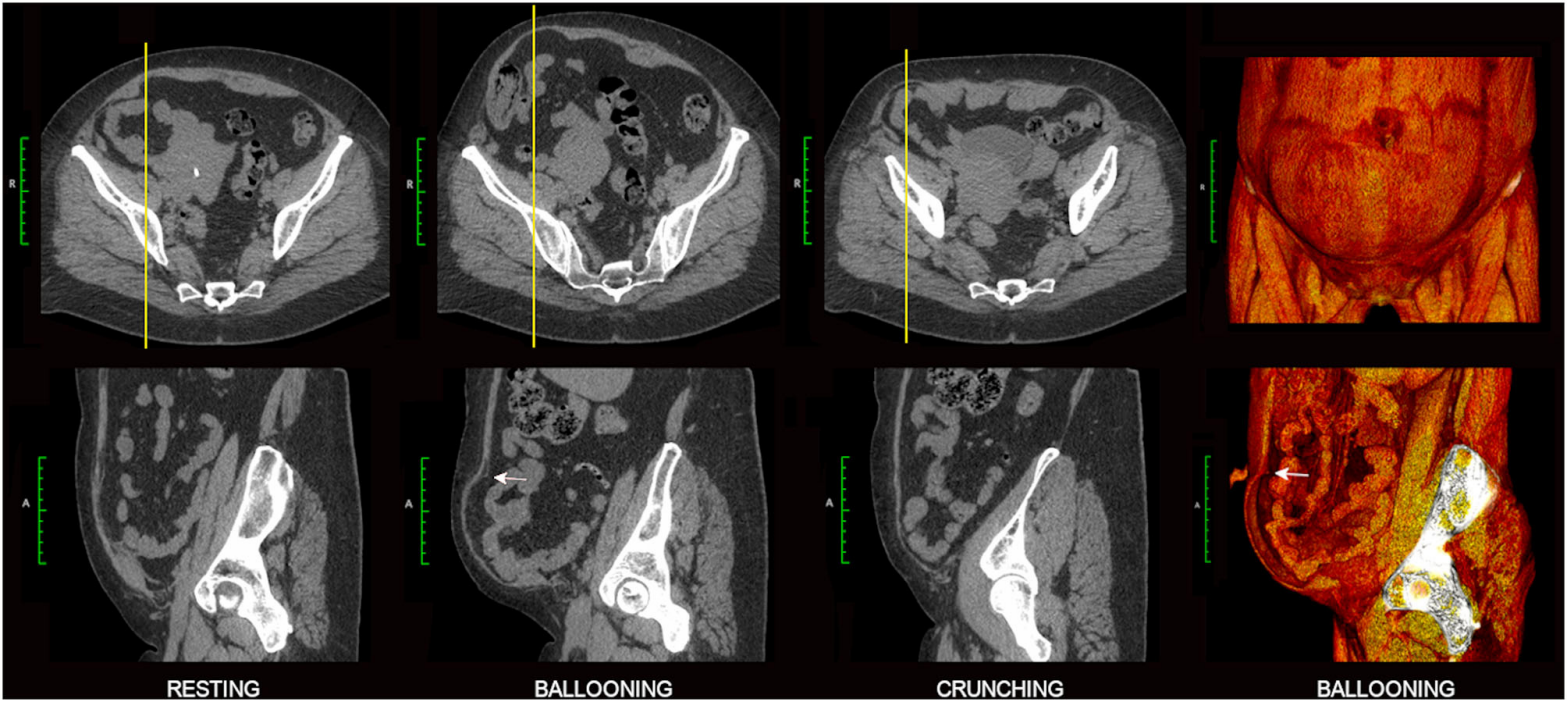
Value of ballooning CT acquisition. Axial and sagittal 2D images are shown from a 3-phase functional CT examination of a 50 year old female with broad-based right lower quadrant incisional hernia. Ballooning images best demonstrate the abnormality with characteristic abrupt “angular shouldering” (white arrows) at the margins of the hernia defect. Companion 3D volume rendered images on the right show the overall extent of lower quadrant bulge (frontal view, top) and profile the rim of the hernia defect (sagittal “cutaway” view, bottom).
